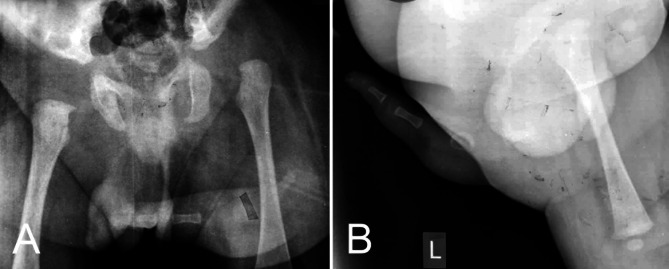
Pelvic X-ray with anteroposterior (A) and lateral (B) views of the accessory limb. The limb does not appear to be articulating with the pelvis. Note the left dislocated hip
